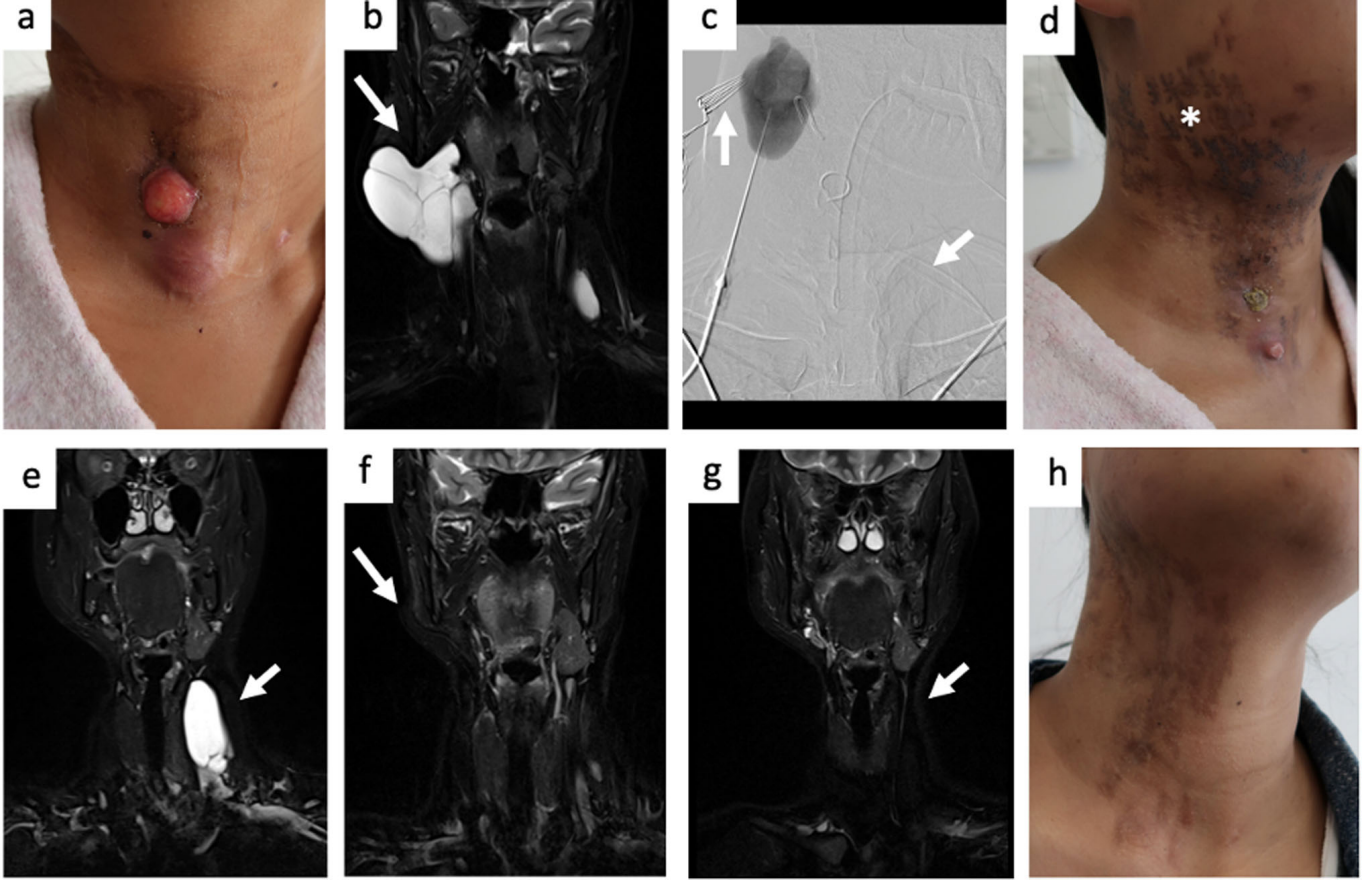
30-year-old female patient with macrocystic lymphatic malformations (LMs) of the neck treated by two sessions of Bleomycin-electrosclerotherapy (BEST) a Clinical photograph before BEST treatment; note the swelling as well as the superficial localized components spontaneously ruptured some weeks before. b Coronal short-tau-inversion recovery sequence (STIR) magnetic resonance images prior to treatment show the extent of the macrocystic LM with predominant component on the right neck as well as smaller component on the left neck both sparing deeper laryngeal areas (arrow) c Periprocedural digital subtraction image during first BEST session, note the puncture of the right neck and filling of macrocystic structures after contrast administration. Partial cyst evacuation to reduce drug dilution and Bleomycin application was performed prior to intralesional electrode placement (arrow). d Clinical photographs 1 month after first BEST session, note the significantly reduced swelling, healing superficial components as well as postprocedural skin discolouration (asterisk). e Coronal STIR magnetic resonance images 6 months after first BEST session revealing complete regression of the right-sided lesion treated as well as persisting LM components left-sided not yet treated (arrow). f, g Coronal STIR magnetic resonance images 6 months after second BEST session revealing near-complete regression of all treated LM components (arrows). h Clinical photograph 6 months after second BEST session, note completely resolved swelling and residual/partially faded postprocedural skin discolourations
